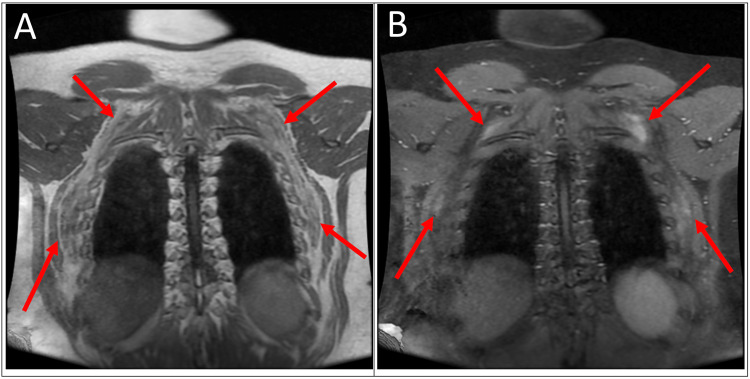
(A) Coronal T1-weighted image demonstrates bilateral suprascapular and bilateral subscapular masses. The masses appear to contain alternating soft tissue and fatty components. (B) T1-weighted post-contrast image with fat saturation showing low-level enhancement.
